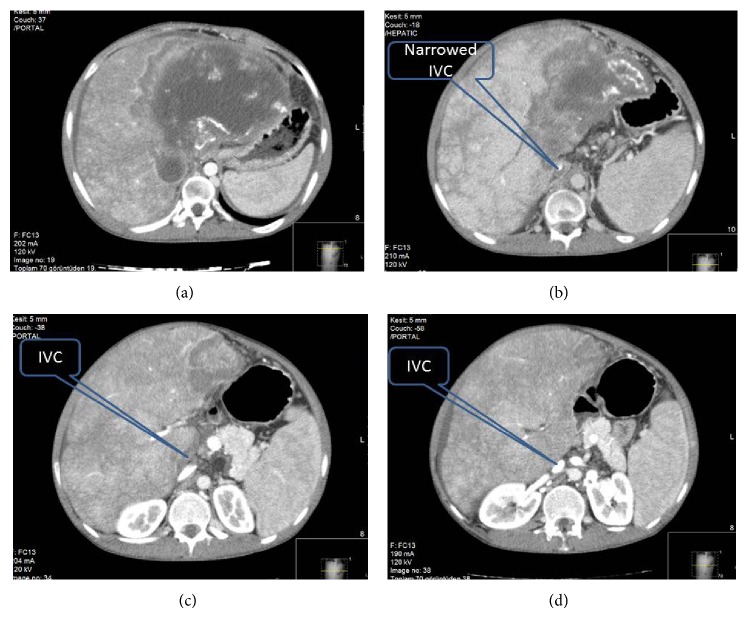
CT view of preoperative liver with (a) large mass filling almost all liver and IVC is nonvisible. (b) Invasion and compression of IVC by EA are seen. (c) Compression to surrounding tissues and organs by EA. (d) Compression and invasion of IVC extending up to renal vein level.
